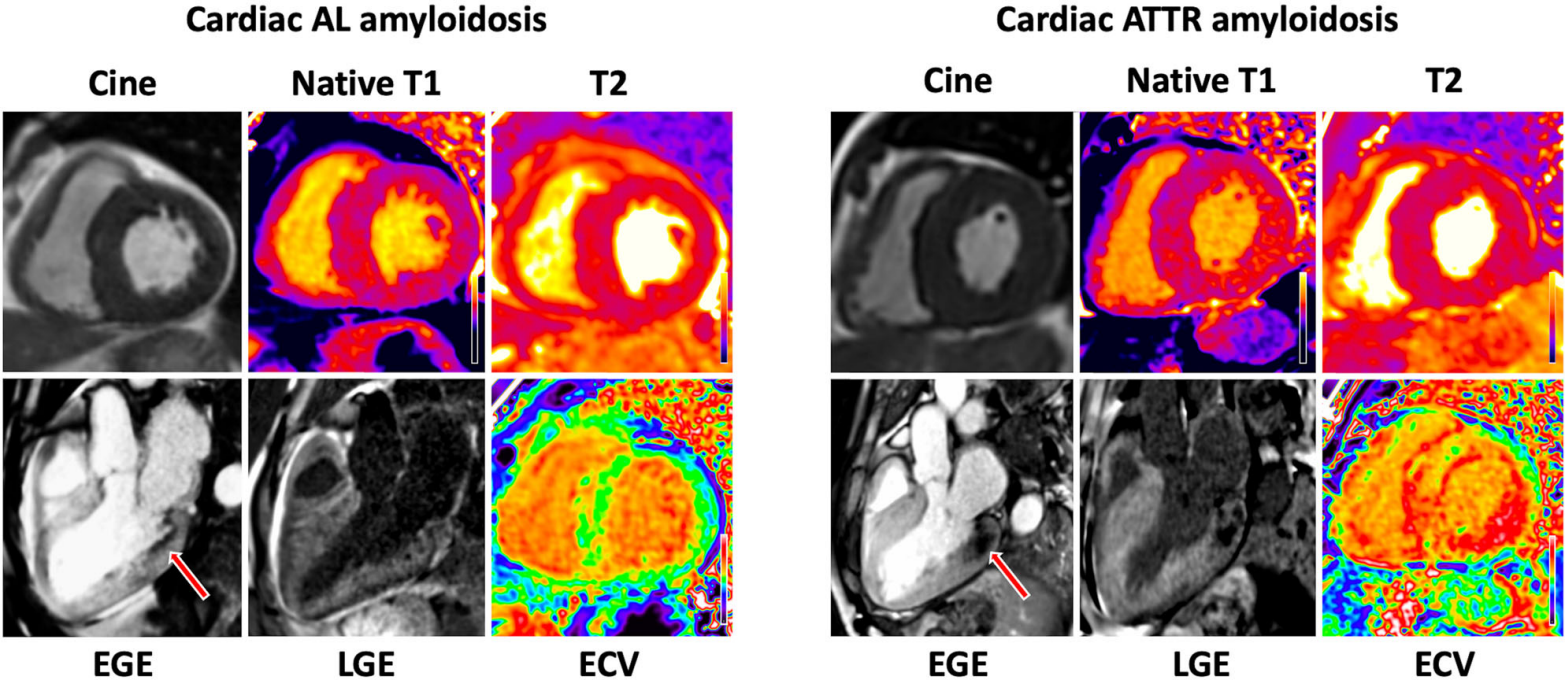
(Left panel) Cardiac magnetic resonance imaging in cardiac light‐chain (AL) amyloidosis demonstrating subendocardial microvascular obstruction (arrow). (Right panel) Cardiac magnetic resonance imaging in cardiac transthyretin amyloidosis (ATTR) demonstrating subendocardial‐to‐midwall microvascular obstruction (arrow). ECV, extracellular volume; EGE, early gadolinium enhancement; LGE, late gadolinium enhancement.
